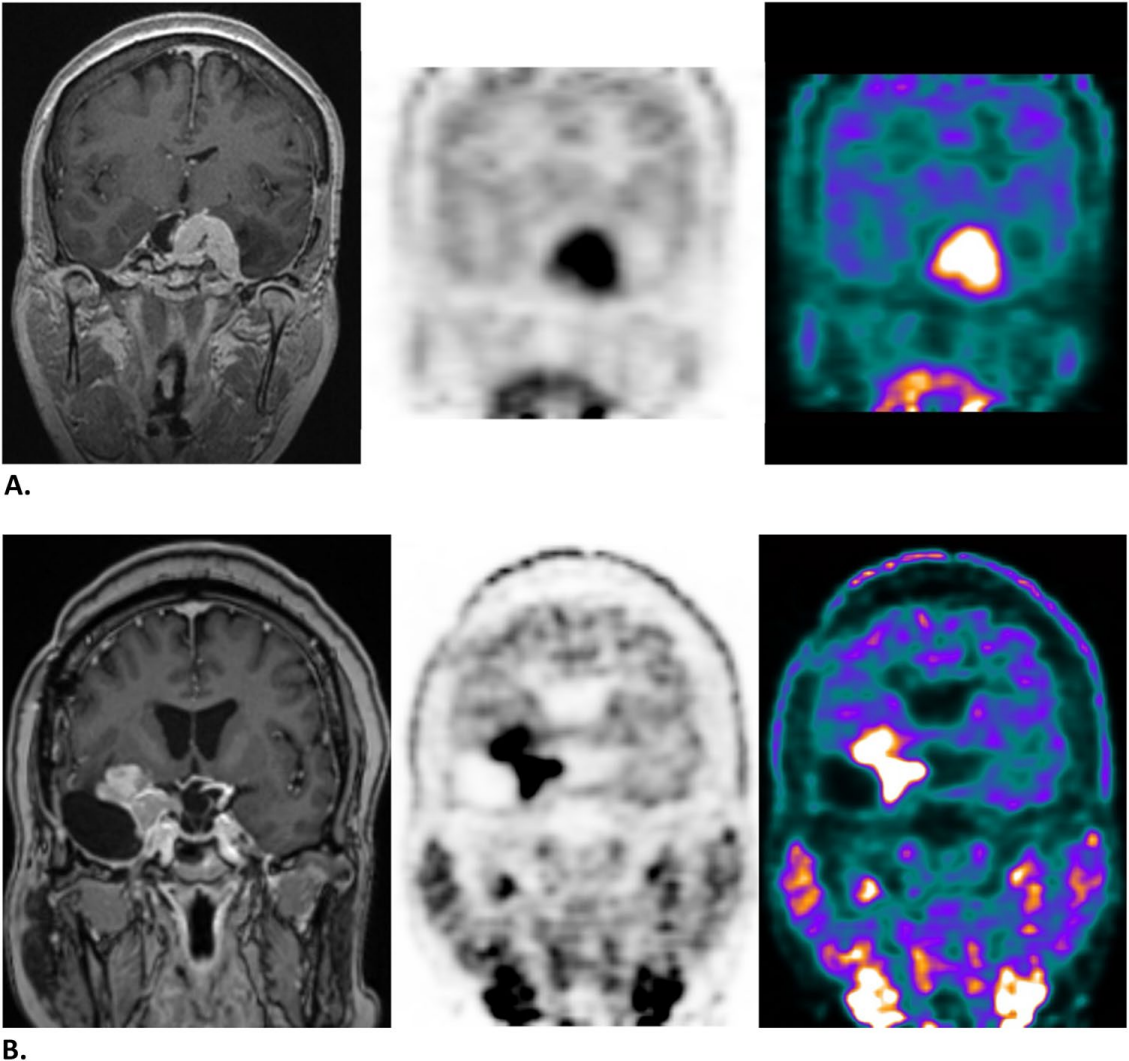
A Coronal T1 gadolinium-enhanced MRI and MET-PET scan of a patient with a meningioma in the left cavernous sinus with stable disease 49 months after SRT. B Coronal T1 gadolinium-enhanced MRI and MET-PET scan of a patient with a right sided sphenoidal meningioma with progressive disease 97 months after SRT and with a history of previous neurosurgical resections before radiotherapy. *MRI* magnetic resonance imaging, *MET-PET*
